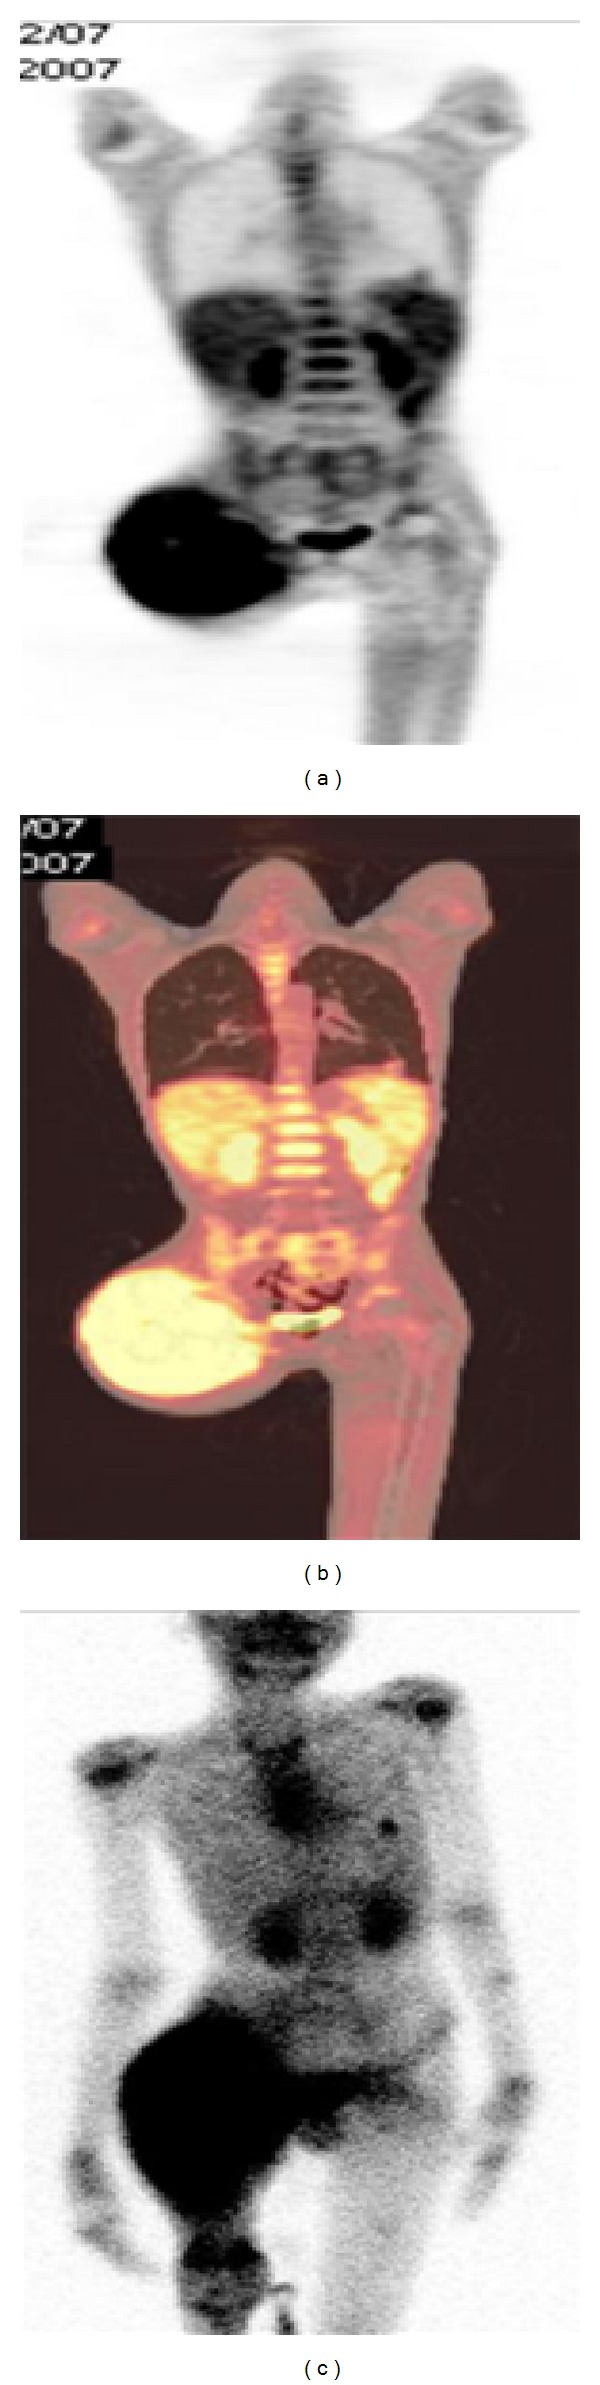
Intense uptake of [^18^F]FDG (a) and ^99m^Tc-DMSA (V) (c) is seen at the primary site in the Rt femur along with the lung nodule in the left lung lower lobe.
